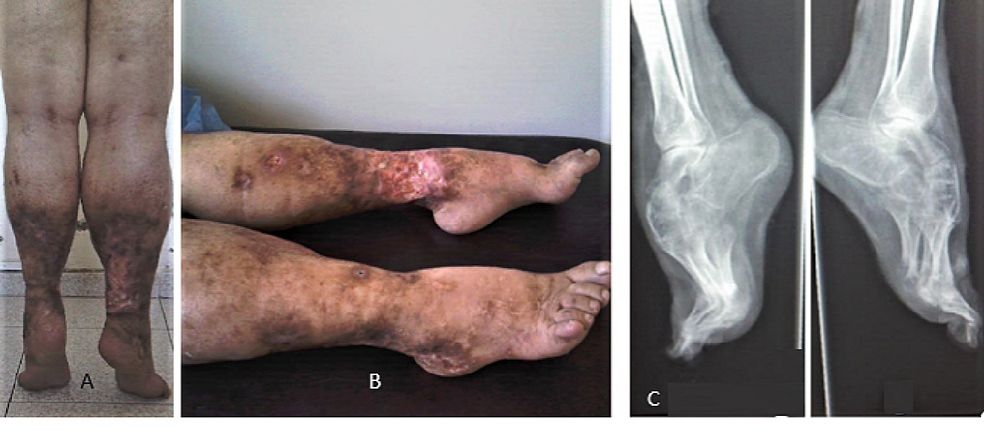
A-B: multiple geographic ulcers on the feet, ankles, and distal tibial areas on both legs, bilateral genu valgum, and bilateral irreducible equinus. C: Lateral foot x-ray: demineralization, and equinus, without joint involvement.
